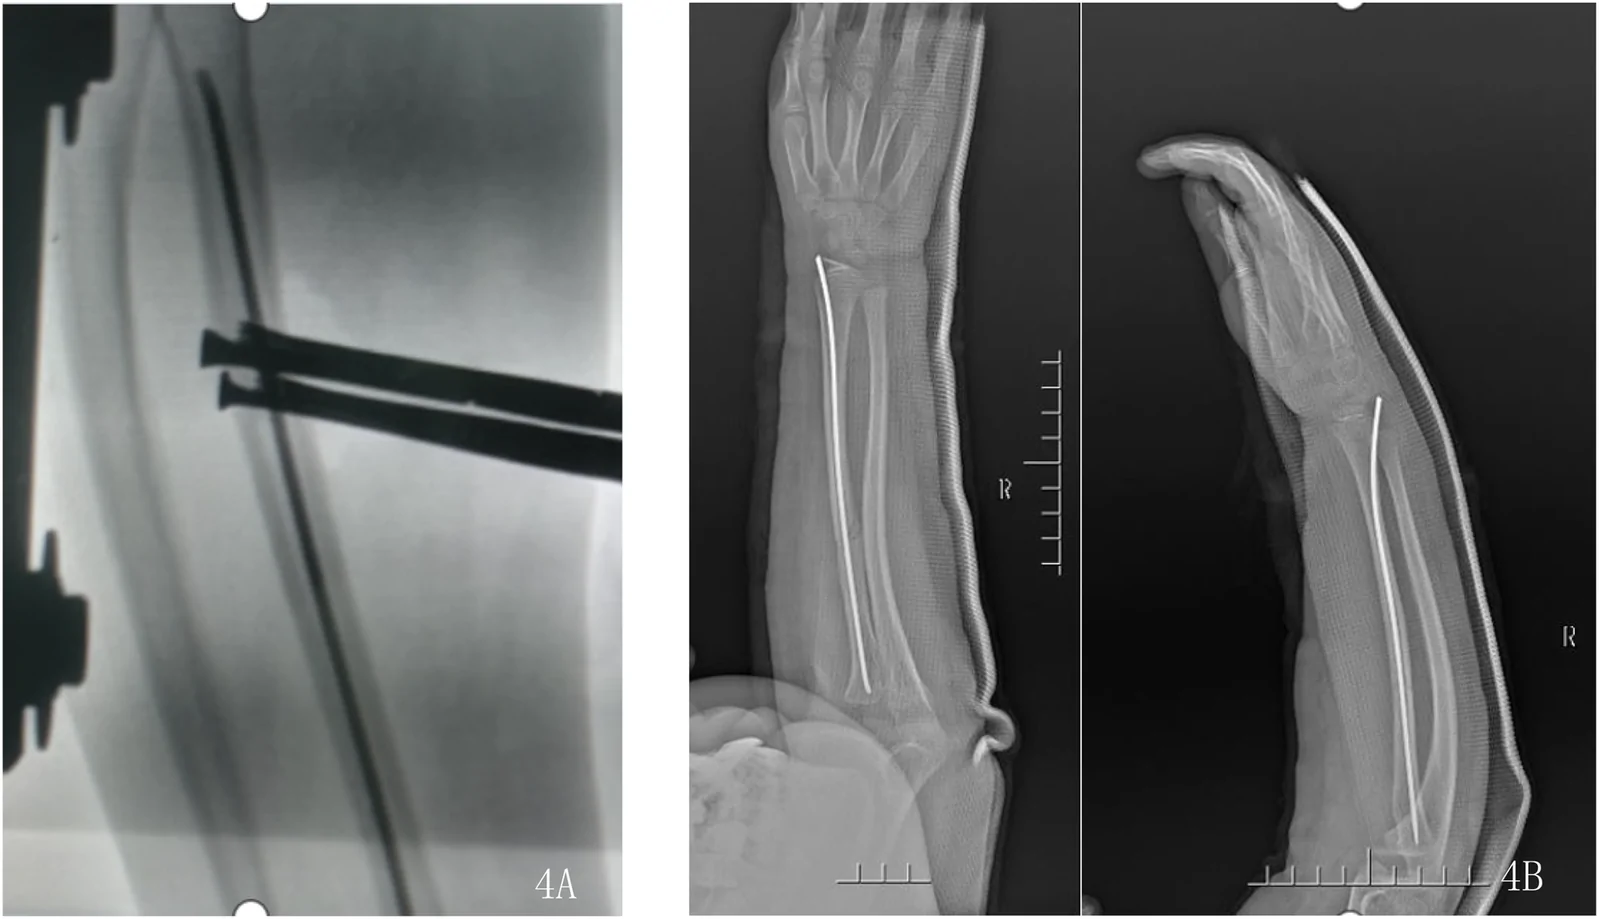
Intraoperative Fluoroscopic view showing anatomical reduction of radial fracture (A); immediate postoperative radiograph confirming proper elastic intramedullary nail placement (B).
